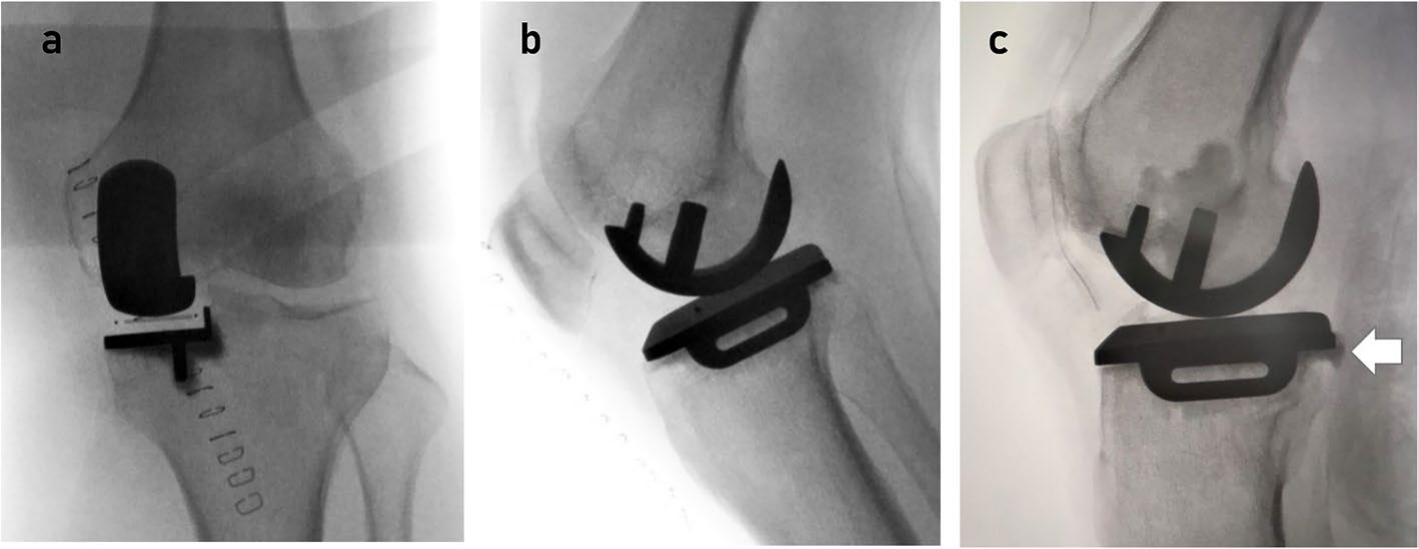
A. The surgeon manipulated X-ray beam and operative limb under fluoroscopic control until the X-ray beam was parallel to the side wall and base plate of the tibial component. B. The patient was positioned in a supine position with the knee flexed 30-40°.The fluoroscope was rotated through 90°so that the X-ray beam was centered on the femoral component. This meant that the X-ray was perpendicular to the side of the femoral component, so as to evaluate the residual cement around the prosthesis. C. One case of fixed excess cement of the posterior tibial component (arrow)
